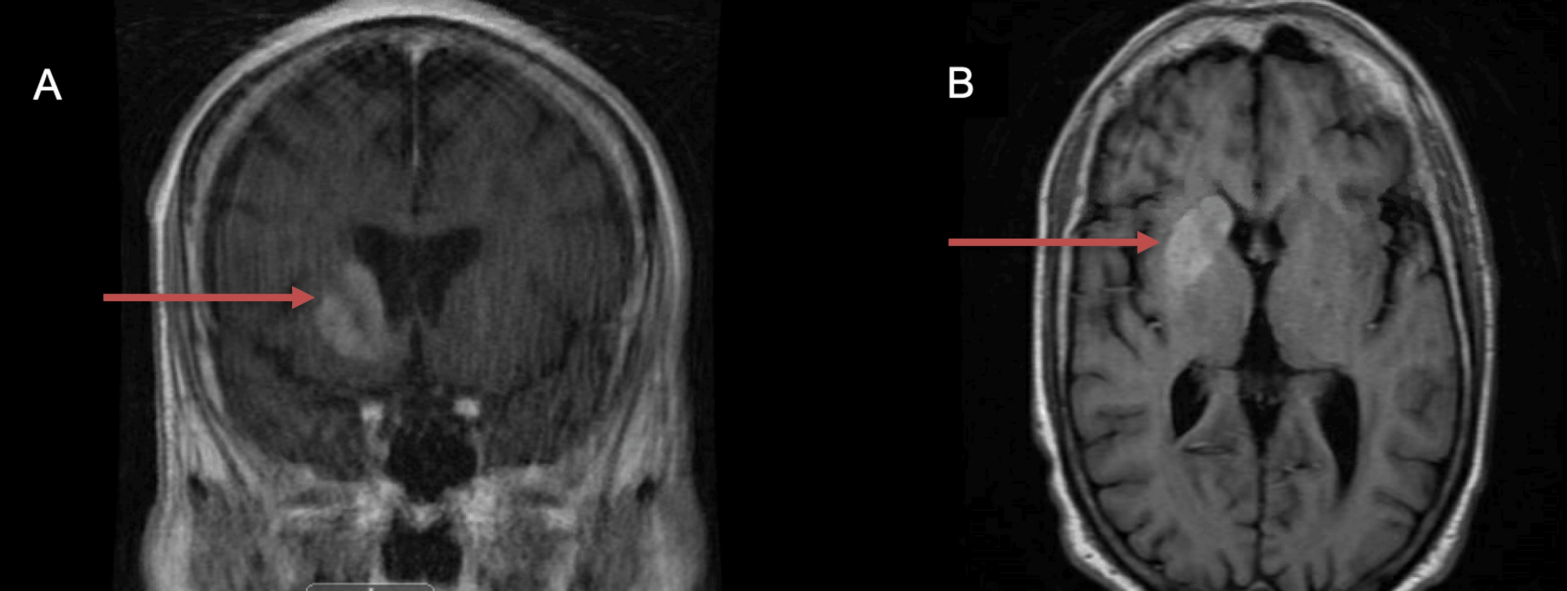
MRI of the brain in coronal (Panel A) and transverse (Panel B) views. Noted increased T1 signal and contrast enhancement (red arrow) involving the right caudate head and the right lentiform nuclei.
